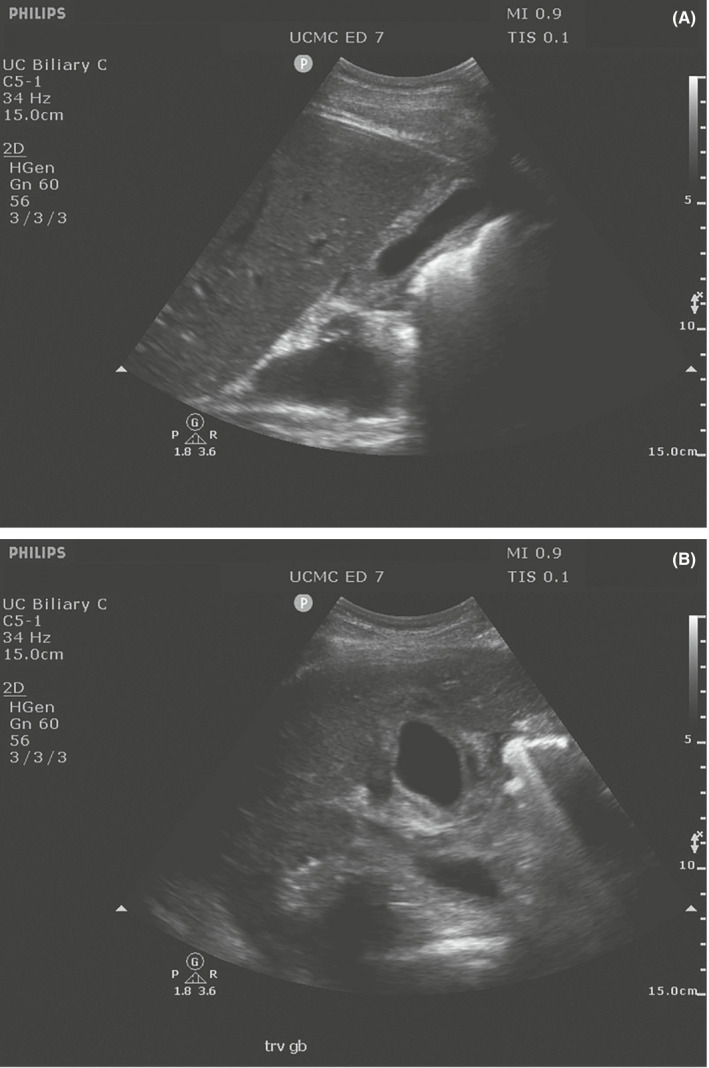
(A) Case 1. Point‐of‐care ultrasound of gallbladder in a longitudinal plane demonstrating a thickened gallbladder wall without any evidence of stones. (B) Case 1. A point‐of‐care ultrasound of the gallbladder in transverse plane demonstrating the double wall sign that can be seen in acute hepatitis. The double wall is a relatively less echogenic area that separates the more echogenic layers of the gallbladder wall
